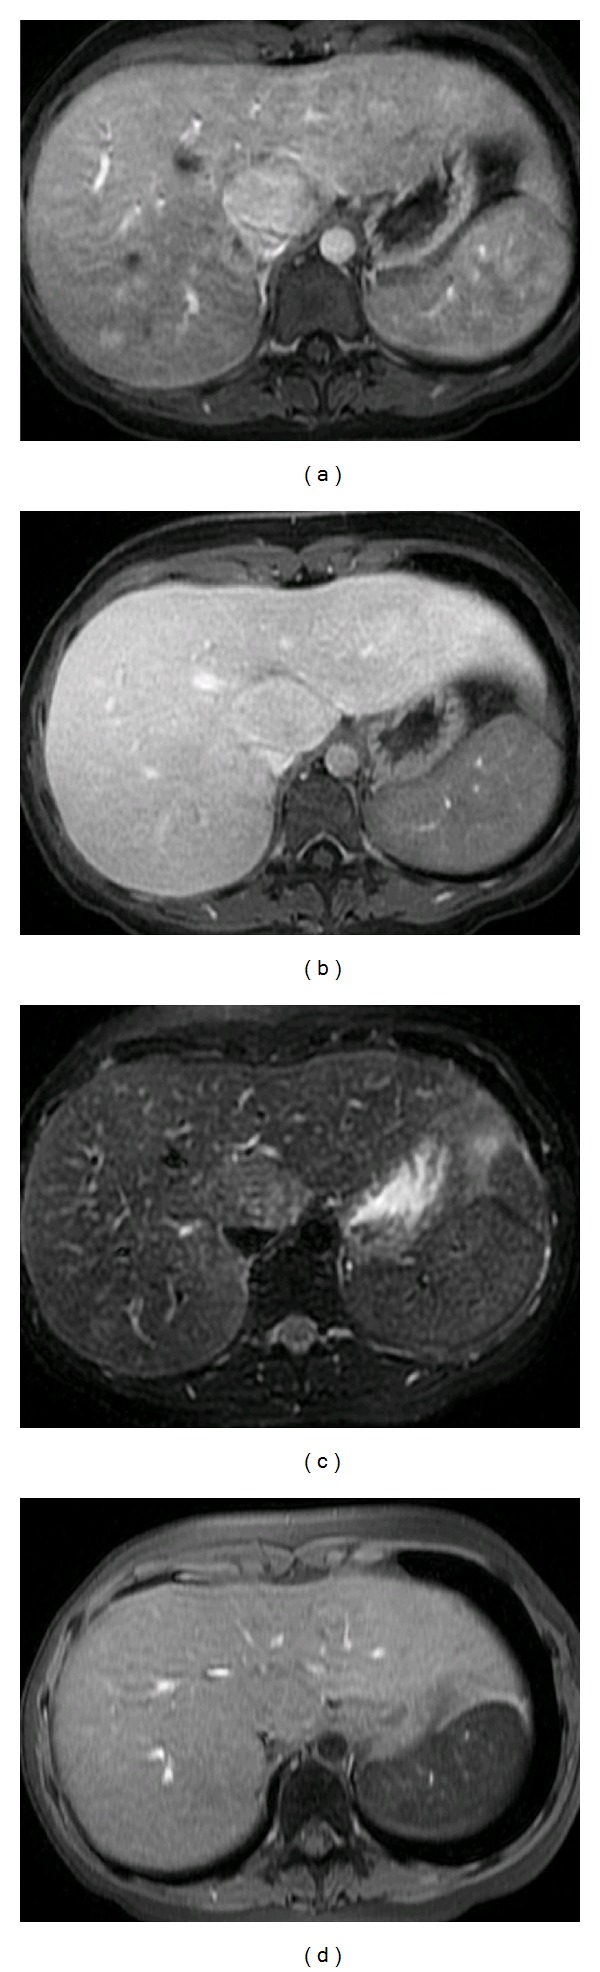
Segment 1 adenoma in gadolinium-enhanced MRI (contrast medium: gadoxetic acid; disodium salt (Primovist, Eurokontrast GmbH, Heidelberg), scanner: GE Signa HDxt 1,5T (General Electric Company, USA)): hyperintens. in T1 FS arterial contrast phase (upper left): (T1 LAVA FS dynamic FA80, TR 185 TE 4,2), partial equilibration to liver isointensity in T1 FS late portal-venous phase (upper right): (T1 FSPGR FS FA12, TR 4,24 TE 2,04, TI 7), slight hyperintensity on T2 FS (lower left): (T2 FRFSE FS FA 90 TR 2500 TE 94,16), and isointensity in unenhanced T1 fat sat. (lower right): (T1 LAVA FS dynamic FA80, TR 185 TE 4,2).
